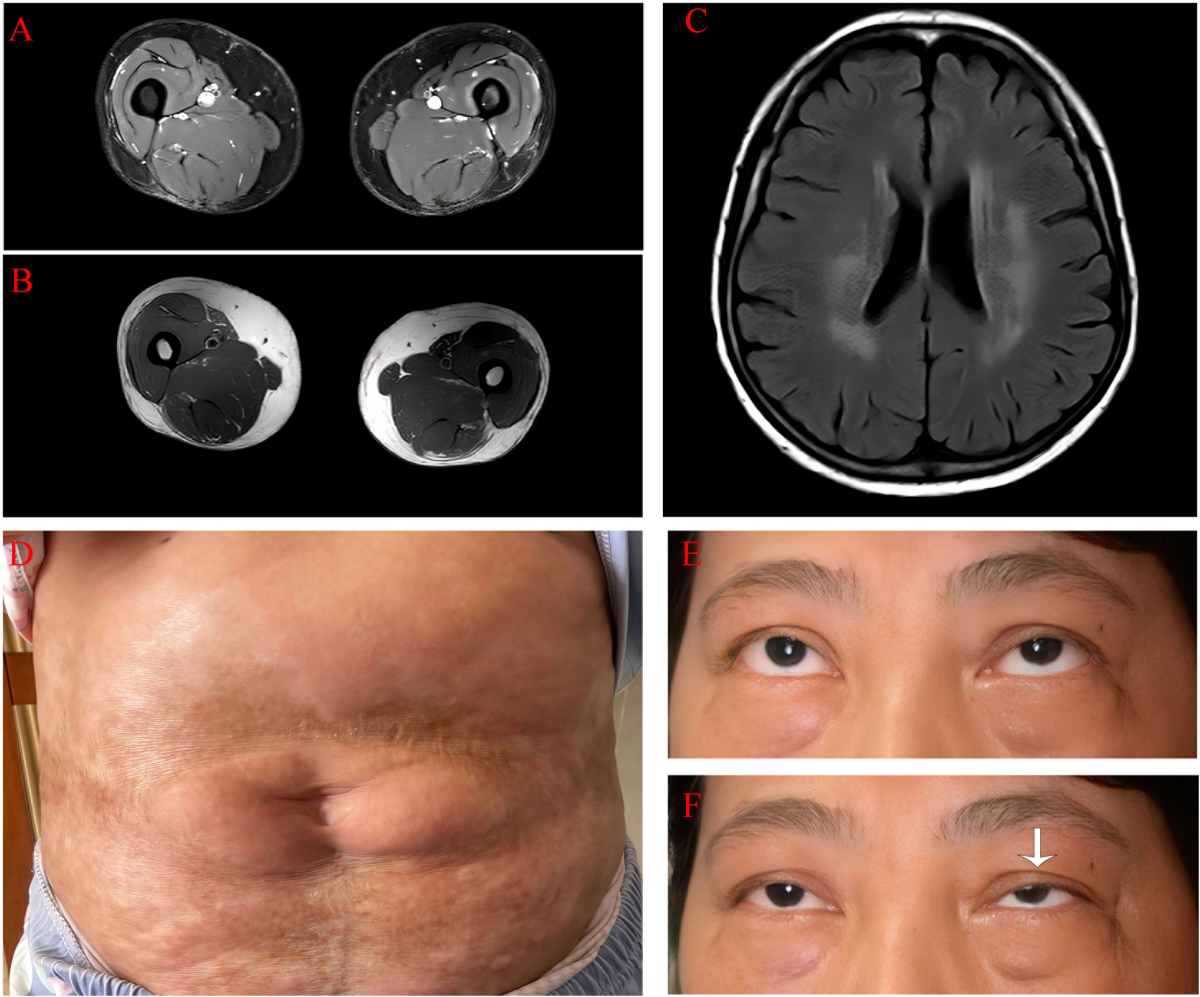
Clinical data of the patient 1. (A) There is no evidence of inflammatory edema in the axial T2 image of the thigh. (B) There is no fibrosis in the axial T1-weighted image of the thigh. (C) Bilateral, patchy, and abnormal signal intensities are observed on plain magnetic resonance imaging of the head, and the fluid-attenuated inversion recovery sequence shows high signal intensity. (D) In the lumbar and abdominal regions, there are observed band-like and patchy distributed areas of sclerotic atrophy of the skin with diffuse hyperpigmentation. The affected areas demonstrate surface depression and partial waxy luster. (E) The patient does not have ptosis. (F) After maintaining upward gaze for 5 minutes, the patient exhibited marked left-sided ptosis (as indicated by the white arrow).
